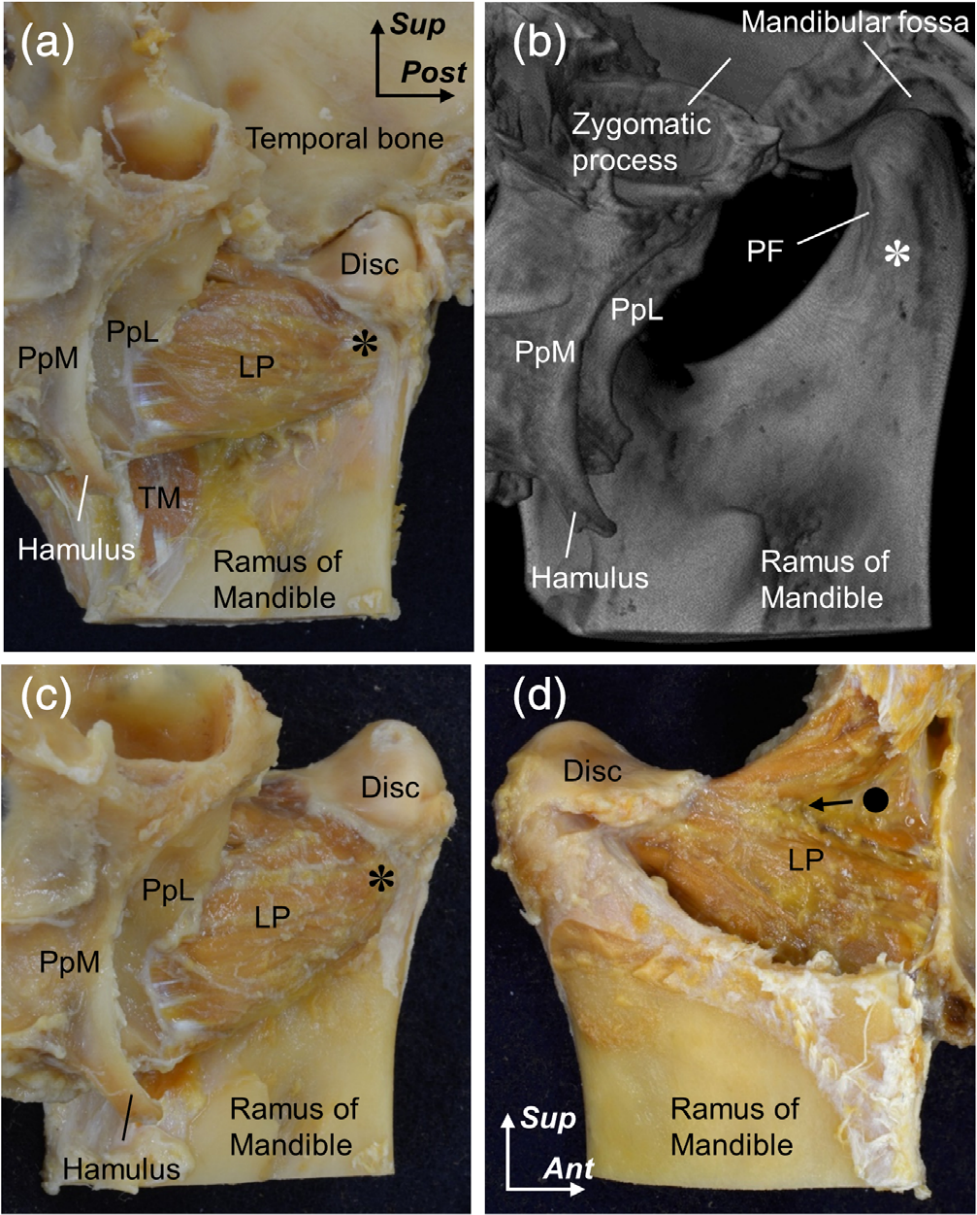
Method of preparation for the detailed mapping of the muscle fiber arrangement. Medial (a–c) and lateral (d) aspects of the right ramus of the mandible. After exposing the medial surface of the lateral pterygoid (LP) and identifying its attachment sites (a), the bony configurations of the attachment sites are examined using micro‐CT (b). The asterisk indicates the medial surface of the condylar process corresponding to the region of LP insertion. After taking micro‐CT, the bony elements (except the attachment sites) are removed to facilitate observation of the muscle fiber arrangement of the medial and lateral aspects of the LPs (c and d). Circle, muscular groove between the upper and lower heads of the LPs; Disc, articular disc of the temporomandibular joint; Hamulus, pterygoid hamulus; PF, pterygoid fovea of the condylar process; PpL, lateral plate of the pterygoid process; PpM, medial plate of the pterygoid process; TM, temporal muscle. *Ant*, anterior; *Post*, posterior; and *Sup*, superior
